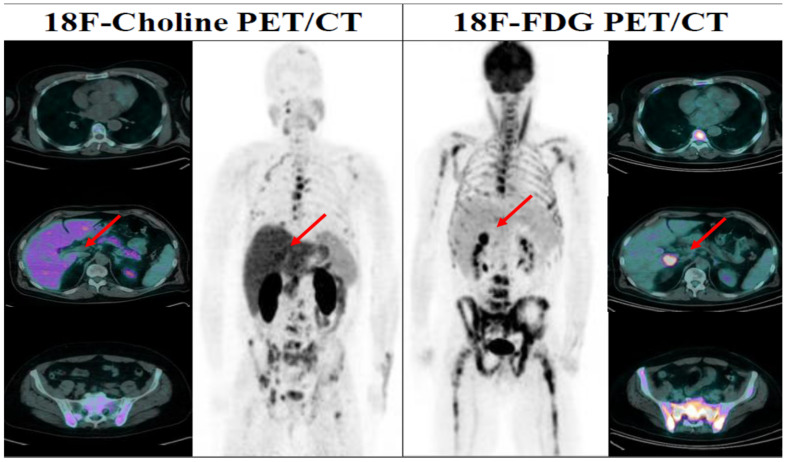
Comparison of 18F-Choline PET/CT and ^18^F-FDG PET; 18F-FDG PET shows high osteo-medullary tracer uptake and a focal area of uptake in the liver (red arrows), while 18F-Choline PET shows only a few bones metastasis.
